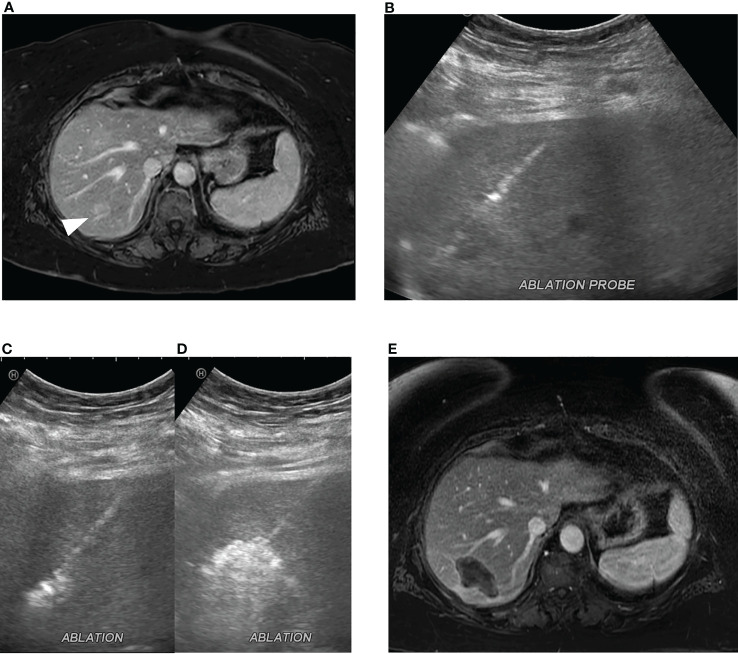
Microwave ablation of colorectal cancer liver metastasis. (A) A 2.0 cm colorectal cancer metastasis in segment 7 (white arrowhead). (B) Ultrasound-guided microwave ablation probe placement in the segment 7 lesion which was confirmed with CT (not shown). Continuous monitoring of ablation was performed with ultrasound with (C) early and (D) late ablation images obtained. (E) Post-ablation MRI, 1 month post procedure, demonstrates ablation zone without evidence of residual disease.
